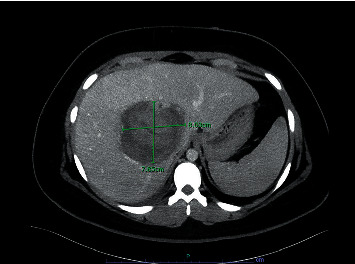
CT scan of the abdomen performed the next day after embolization showing the decrease in the dominant lesion size to 8.0 × 7.9 cm.
